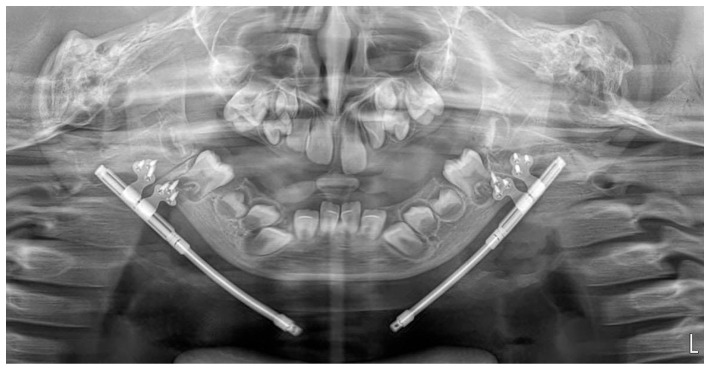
Panoramic radiograph performed on the first postoperative day showing appropriate positioning of the osteotomy line and an adapted distractor.
